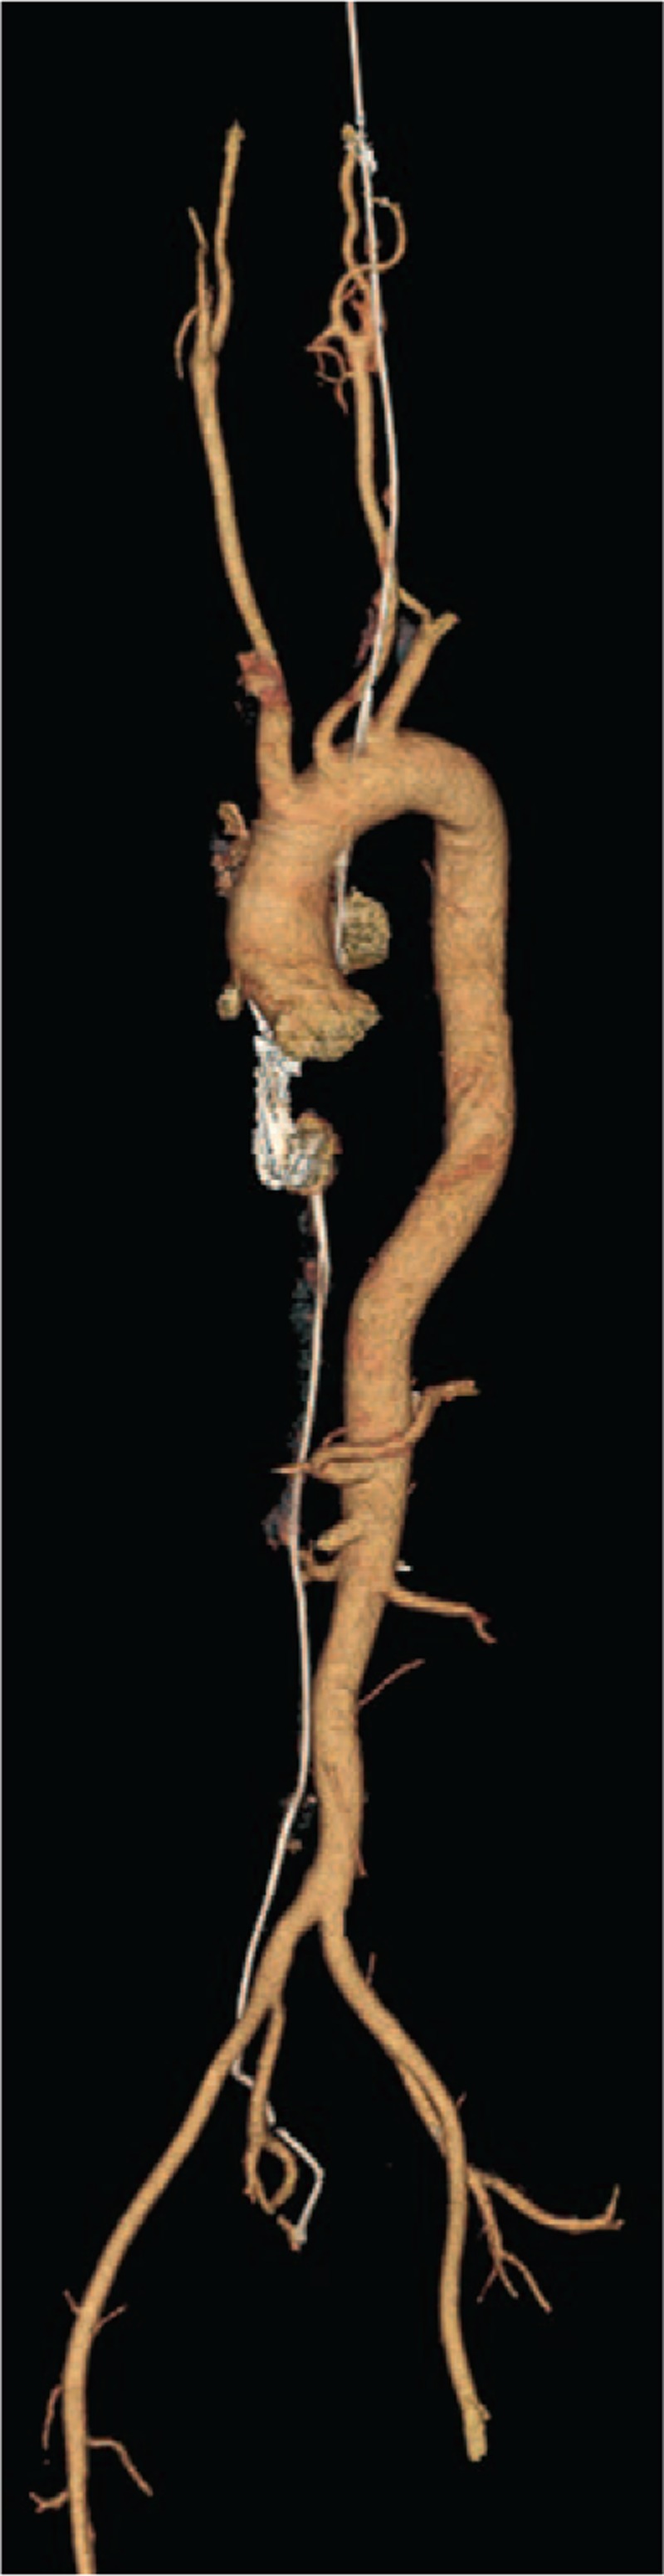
Computed tomography angiography showing guide wire had punctured the vascular wall of the aortic arch to reach the cerebrum.
